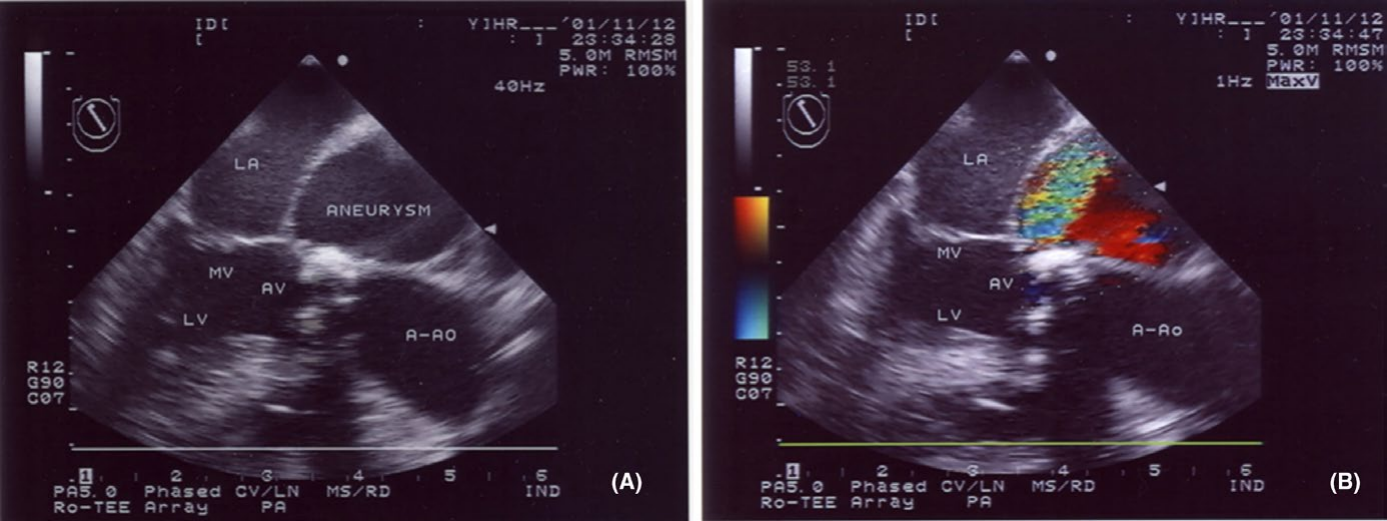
A, Transesophageal echocardiographic image (long‐axis view) of the left ventricular outflow tract showing a left ventricular aneurysm adjacent to the left atrium. B, Transesophageal echocardiographic image, same view as in image A, but with color‐flow Doppler added. Flow is clearly seen from the left ventricle to the aneurysm. A‐Ao, ascending aorta; AV, aortic valve; LA, left atrium; LV, left ventricle; MV, mitral valve
